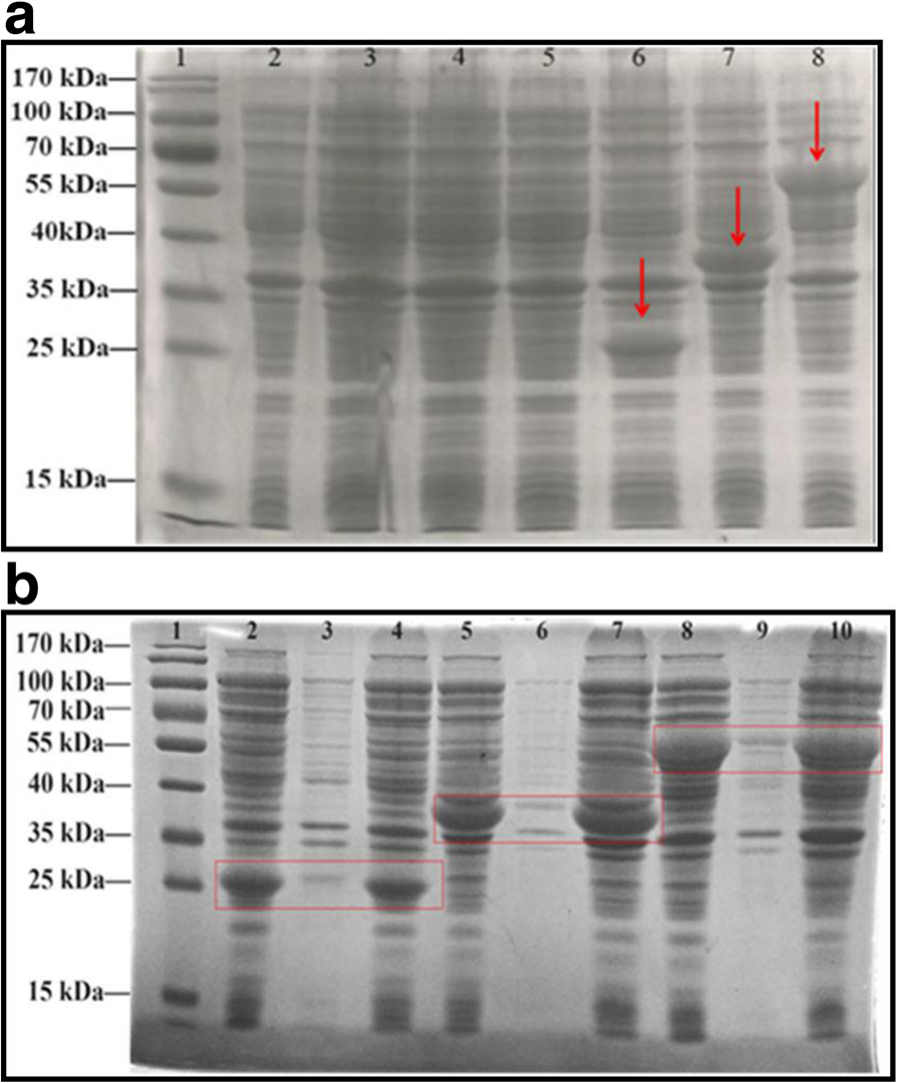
Expression and solubility analysis of hELPs-RADA-16 expressed in BL21 (DE3) by SDS-PAGE. a Expression of hELPs-RADA-16 expressed in BL21 (DE3). Lane 1: Protein marker; Lane 2: IPTG-induced cells transformed by pET28a (+); Lane3–5: cell lysis of uninduced cells transformed by expression vectors of 36R, 60R and 96R, respectively; Lane 6–8: cell lysis of IPTG-induced cells transformed by expression vectors of 36R, 60R and 96R, respectively. b solubility analysis of hELPs-RADA-16. Lane1: Protein marker; Lane 2–4: supernatant, precipitate and total proteins of ultrasonic cracking cell lysis of BL21 (DE3) transformed by pET28a (+)-hELP36-RADA16; Lane5–7: supernatant, precipitate and total proteins of ultrasonic cracking cell lysis of BL21 (DE3) transformed by pET28a (+)-hELP60-RADA16; Lane8–10: supernatant, precipitate and total proteins of ultrasonic cracking cell lysis of BL21 (DE3) transformed by pET28a (+)-hELP96-RADA16
